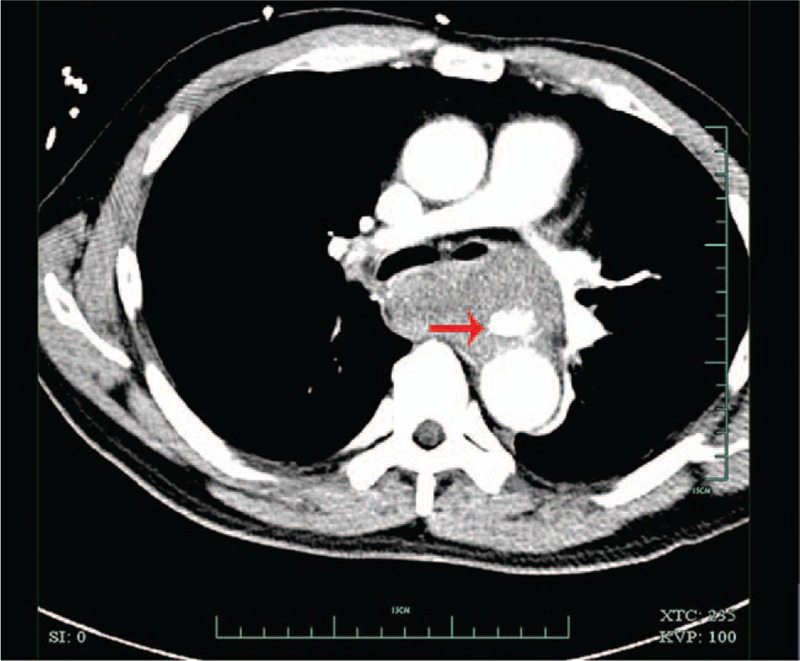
Thoracic aorta computed tomography scan before surgery. Remarkable aneurysm was detected in the thoracic aorta, which ruptured into the mediastinum (red arrow).
